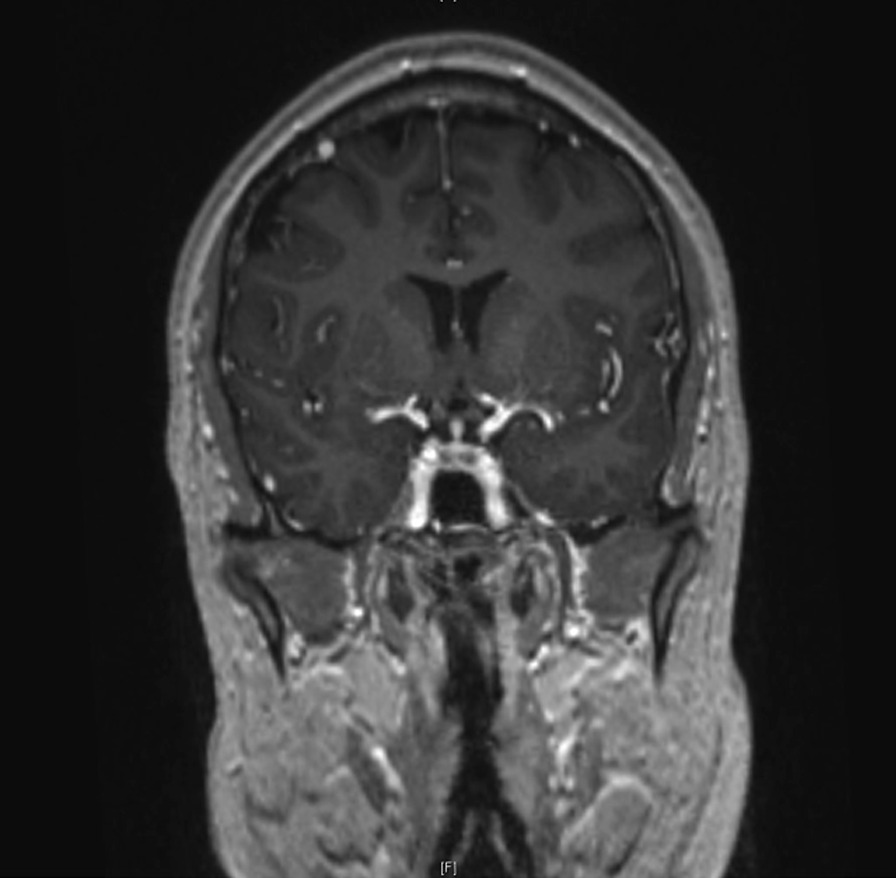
Coronal imaging of the hypophysis on a 3-T T1-weighted MRI image
